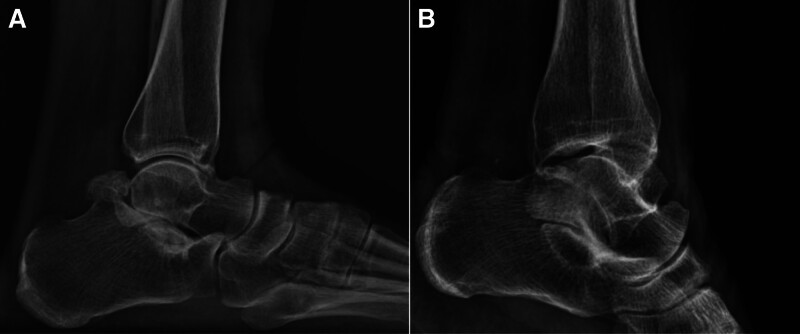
(A) Preoperative lateral radiograph showing a bipartite talus. (B) Postoperative lateral radiograph showing the bipartite talus removed.
